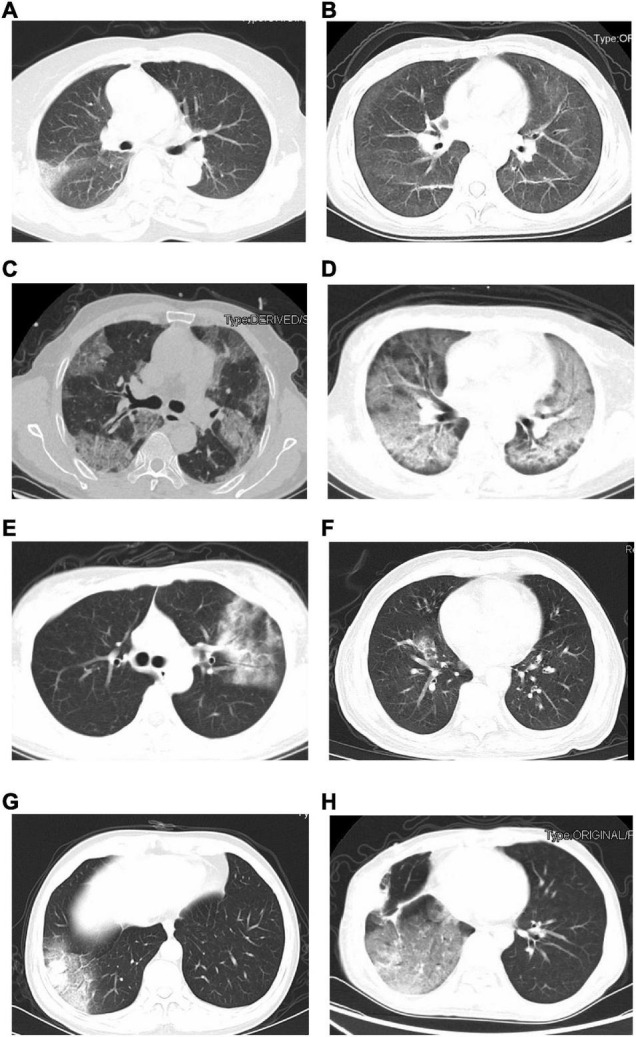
The initial chest CT features of SARS-CoV-2-Negative community acquired pneumonia patients (emergent viruses positive patients: A–D and emergent viruses negative patients: E–H). (A) Ground-glass opacities in right lung (bacterial pneumonia). (B) Ground-glass opacities, strip shadow, and partial interlobular septal thickening in double lung (AIDS). (C) Ground-glass opacities and nodules were widely distributed in both lungs, Crazy-Paving sign could be seen in some lesions (AIDS). (D) Diffuse distribution of patchy shadows, reticulation and multiple nodules in both lungs (systemic lupus erythematosus, lupus nephritis, and pneumocystis pneumoniae). (E) Ground-glass opacities, reticulation, and consolidation in left lungs (*Chlamydia pneumoniae* pneumonia). (F) Ground-glass opacities and consolidation in right lung (*Mycoplasma pneumonia*). (G) Ground-glass opacities and consolidation in right lung (alcoholic cirrhosis and bacterial pneumonia). (H) Chest CT scan shows Ground-glass opacities in right lungs (postoperative lung cancer, immune-associated pneumonia, and presumptive bacterial pneumonia).
